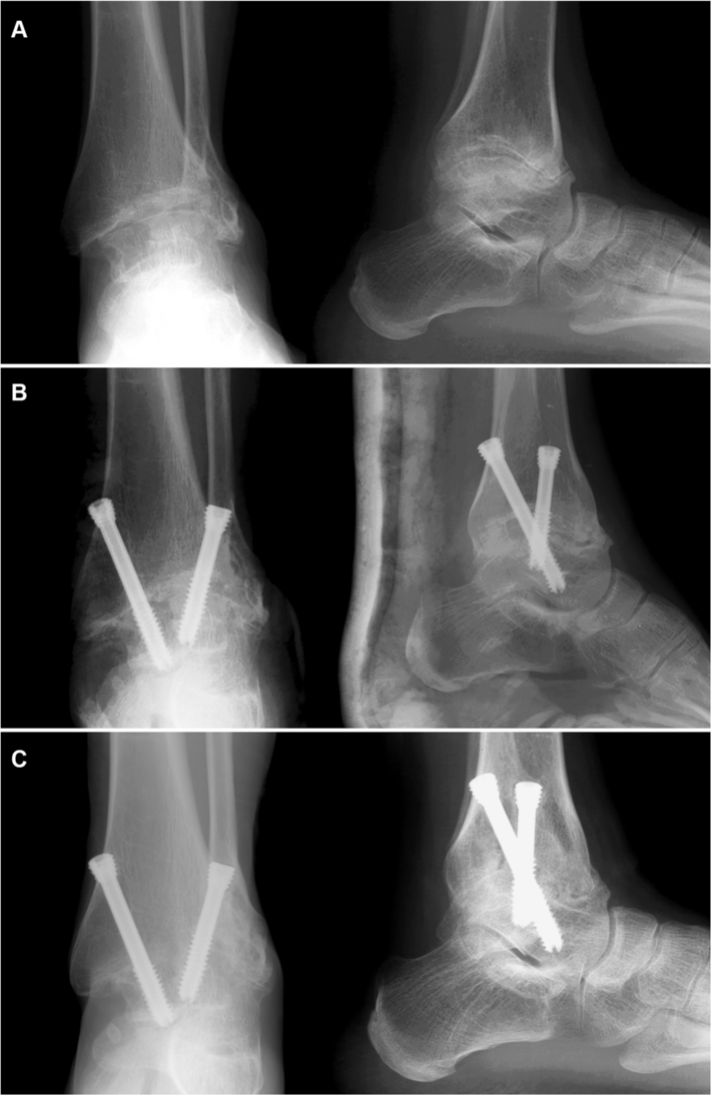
Case 1. a Preoperative radiographs. b Radiographs at 1 day postoperatively. c Radiographs at 4 years postoperatively
